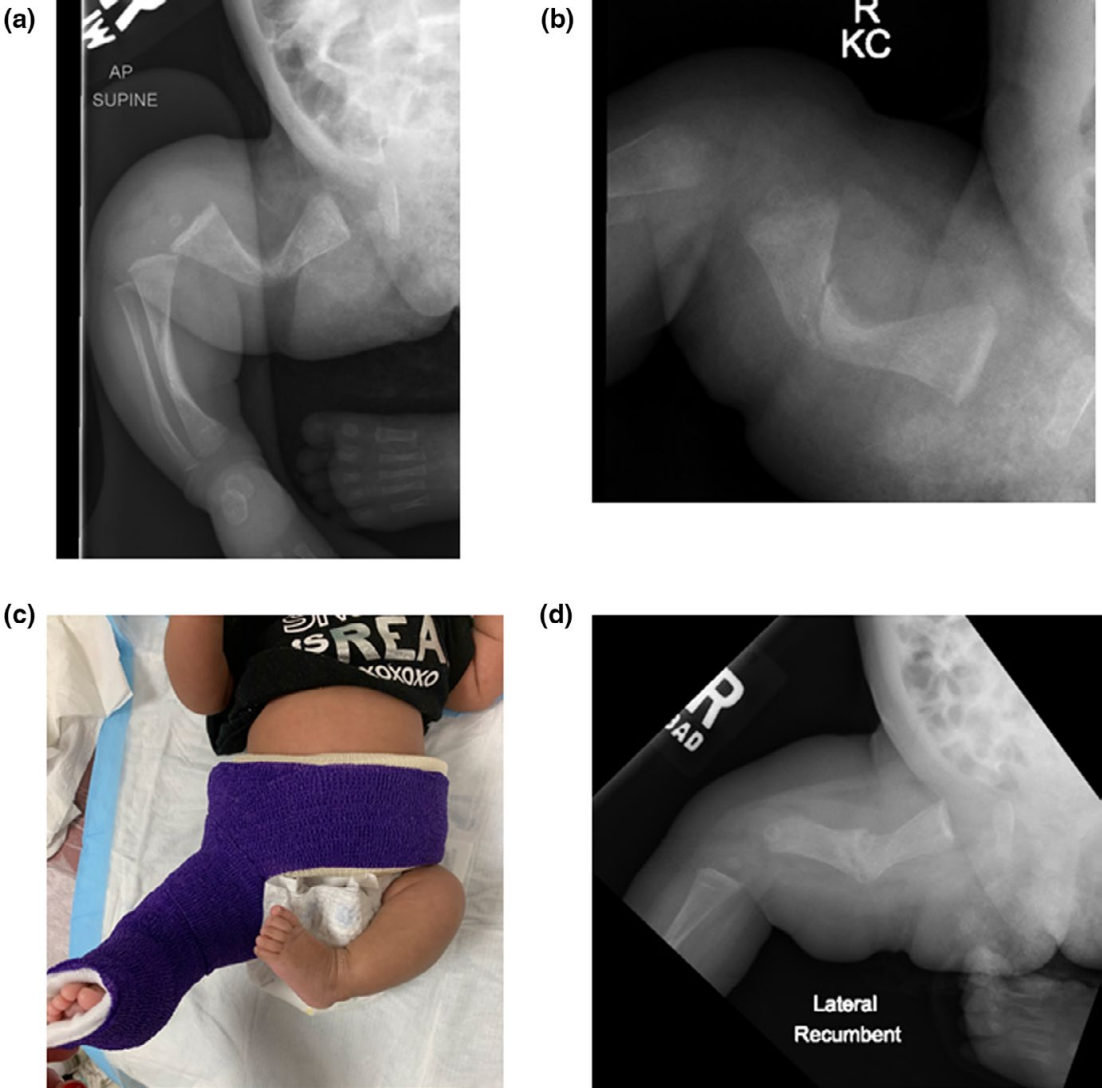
a & b, Two views of the right femur in a 20‐day‐old girl with moderate osteogenesis imperfecta and a right femur fracture. c, Soft wrap immobilization with overlying flexible cohesive bandage and a foam wrap around the lower trunk to complete a soft spica wrap. d, Right femur radiograph 4 weeks later when soft spica wrap was removed
